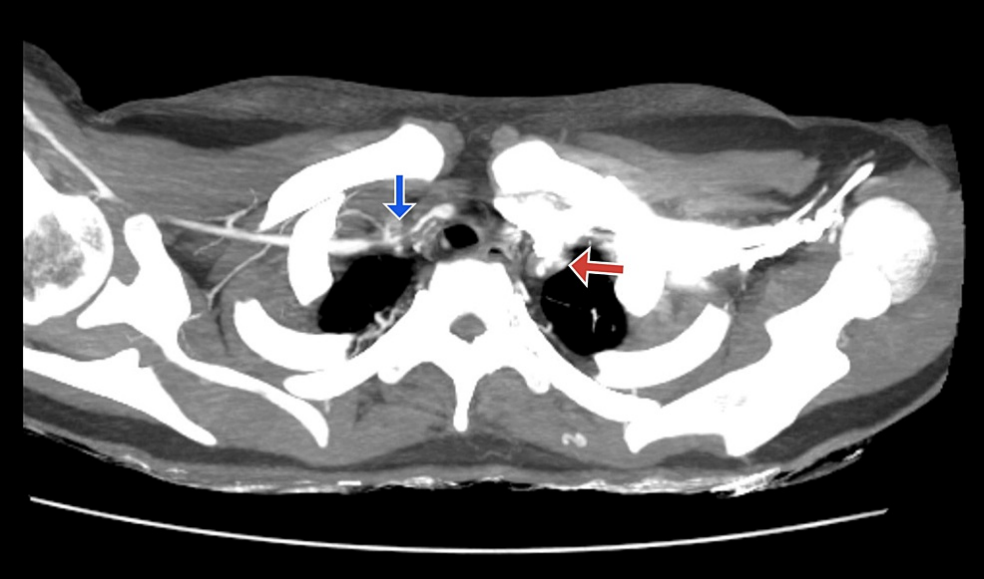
CT aortogram with the blue arrow showing the narrowing of the brachiocephalic trunk and the red arrow showing the narrowing of the left subclavian artery
